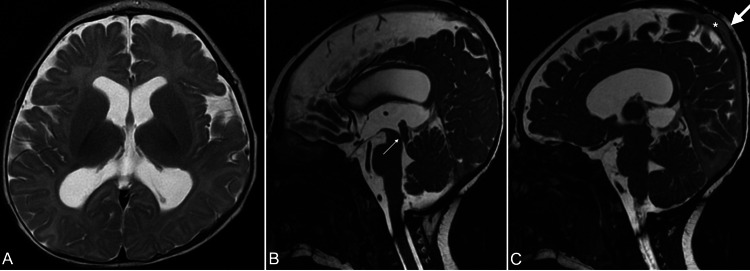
The magnetic resonance imaging of a four-month-old girl with cranium bifidum occultum The axial T2-weighted MRI demonstrates a mild dilation of lateral ventricles, with no enlargement of the third ventricle (A). The sagittal T2-weighted MRI depicts a narrow cerebral aqueduct (thin white arrow), and the parasagittal T2-weighted MRI reveals a small bone defect (thick white arrow) above the sagittal sinus (*), corresponding to cranium bifidum occultum.
